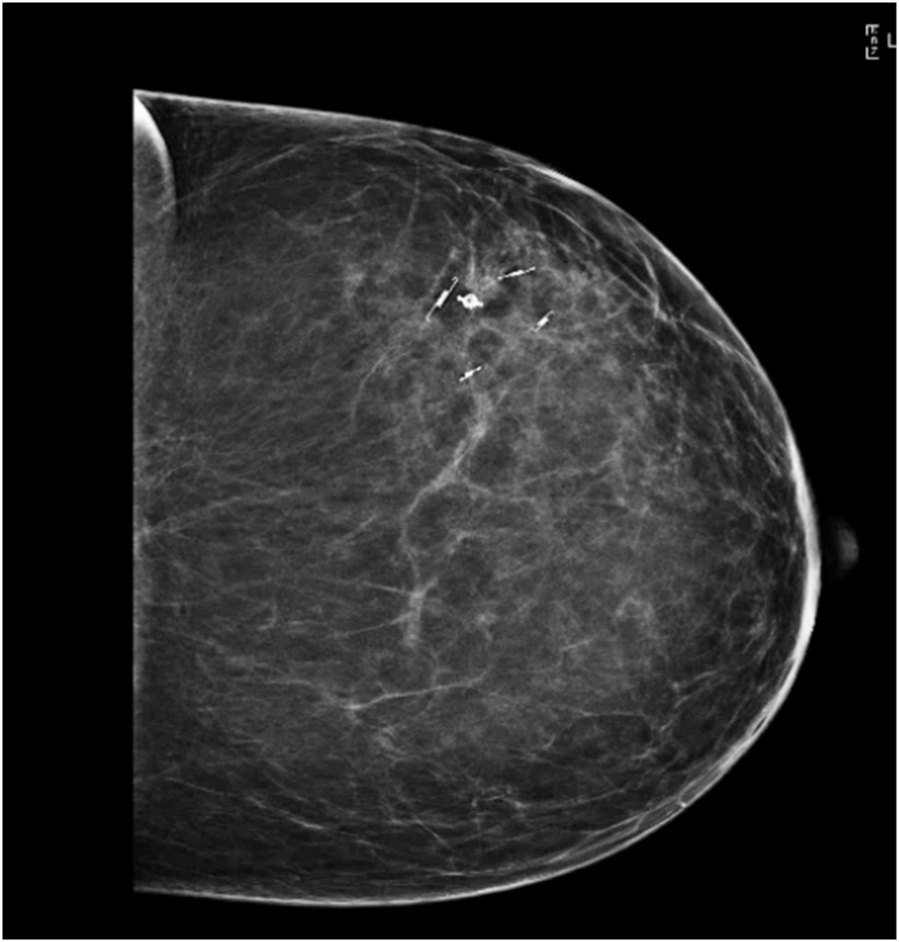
Ultrasound confirmation of SCOUT reflector locations around the calcified lesion. Calcifications surrounded by a double L shape proximally, double J shape laterally, double J shape medially, and JL shape distally.
